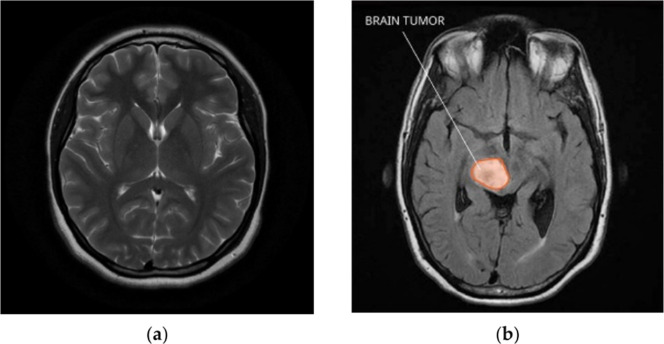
MRI images of brain with (a) No Tumor and (b) Tumor [9].
